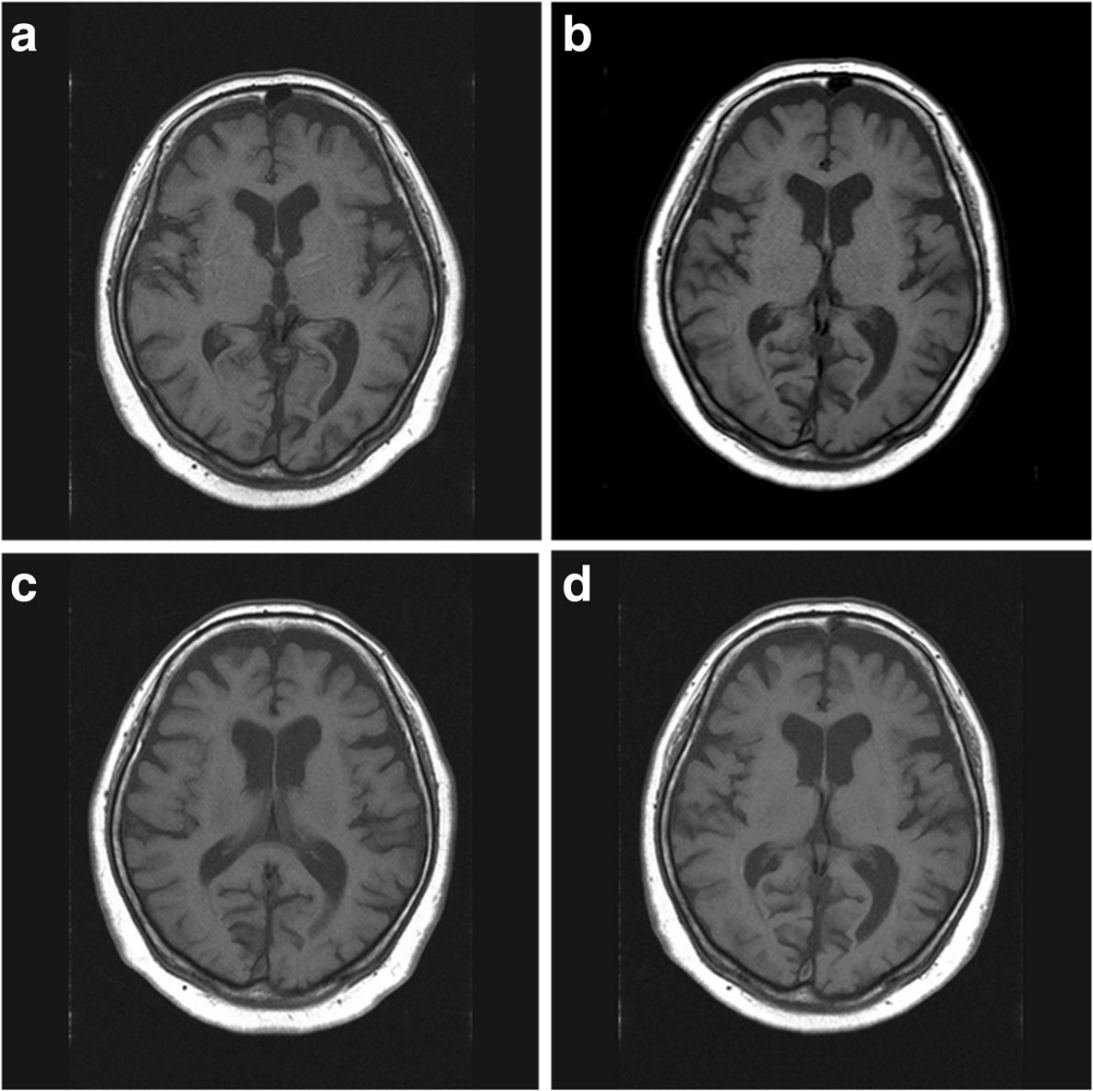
T1-weigted brain MRI scans. a on the 19th hospital day during the first admission. b at 1 month after discharge. c on admission for the second hospitalization. d at the time of hemichorea recurrence. There were no remarkable changes in basal ganglia on any of the T1-weighted brain MRI scans
